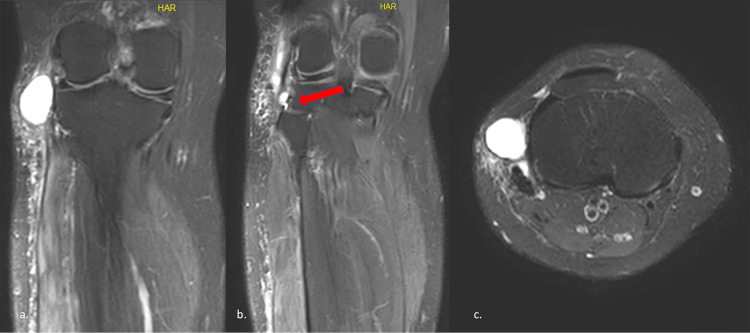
(a) and (b) Preoperative coronal MRI section showing cyst recurrence. Red arrows indicates the PTFJ connection; (c) Axial MRI section of ganglion cyst recurrence PTFJ: proximal tibiofibular joint
